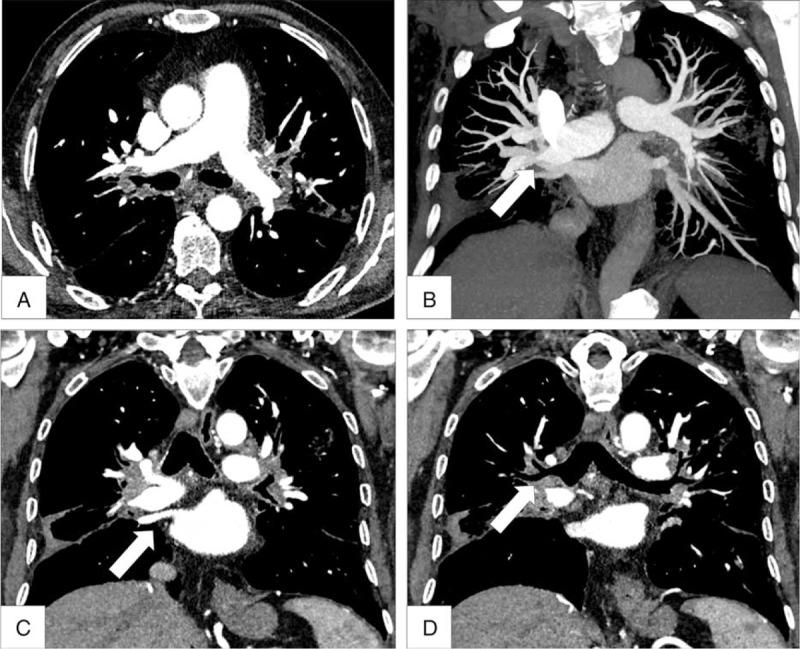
Contrast-enhanced high-resolution computed tomography of the chest of a patient with pulmonary hypertension complicating fibrosing mediastinitis. Panels A and B: Cross-sectional and coronal views showing extrinsic bilateral main pulmonary arteries compression by fibrosing mediastinitis, Panel C: Coronal view showing extrinsic venous compression, Panel D: Coronal view showing bilateral bronchial compression.
